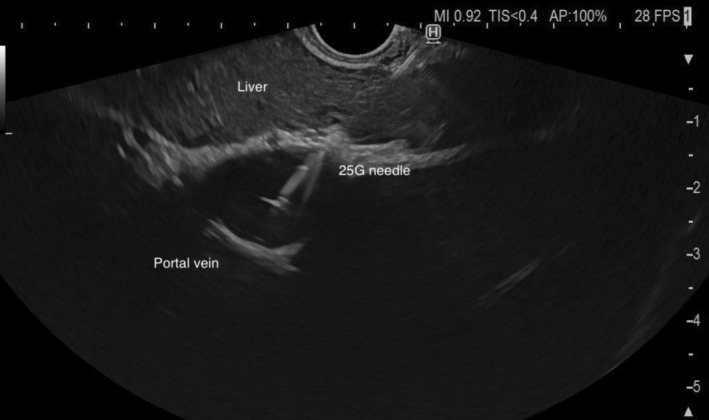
Endoscopic ultrasound‐guided measurement of portal vein pressure.
